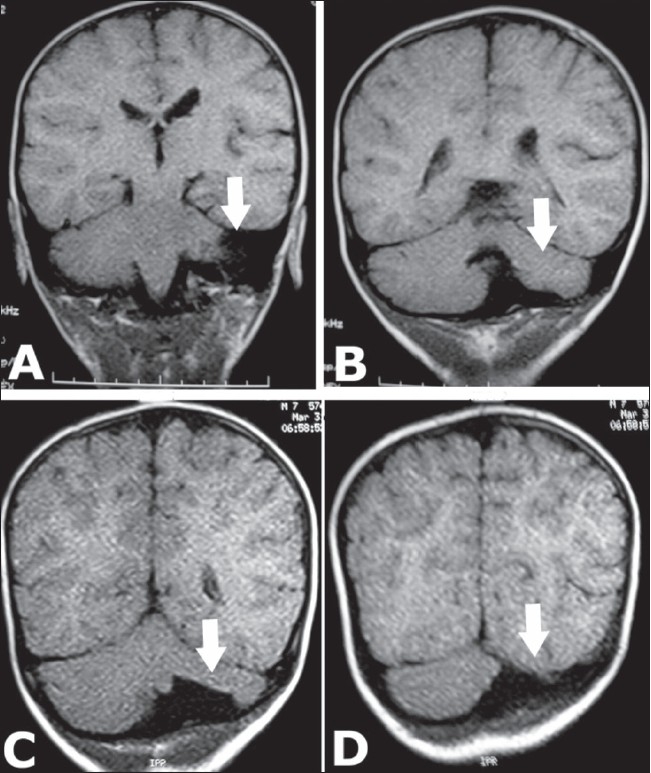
T1W coronal MRI images show left cerebellar hypoplasia (arrows)
